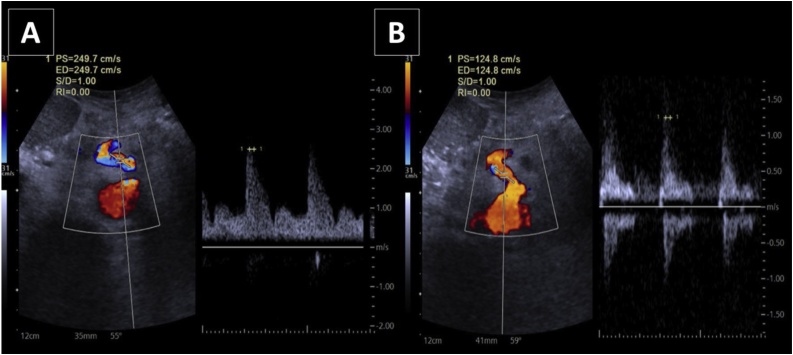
Spectral Doppler US with patient in supine decubitus, during inspiration (3A) and expiration (3B) at the narrower point of the celiac axis color aliasing point. Significantly elevated peak systolic velocity (249.7 cm/s) is seen on expiration with aliasing artifact at color-Doppler mode, 2 times greater than the velocity seen on inspiration (124.8 cm/s).
